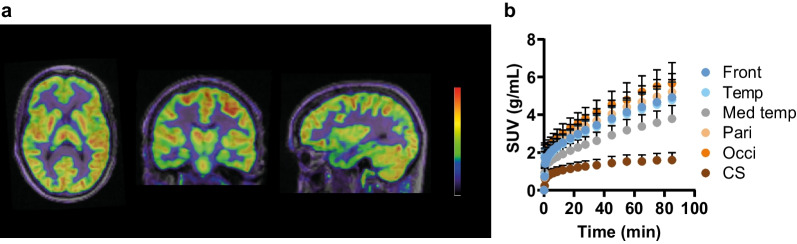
a An [^11^C]UCB-A PET image (averaged 0–90 min), expressed in standardised uptake values (SUV), in a healthy volunteer. The PET image is fused with the MR image from the subject. b Mean time-activity curves (TACs) based on SUV in the frontal lobe (Front), temporal lobe (Temp), medial temporal lobe (Med temp), parietal lobe (Pari), occipital lobe (Occi), and centrum semiovale (CS) from all subjects
